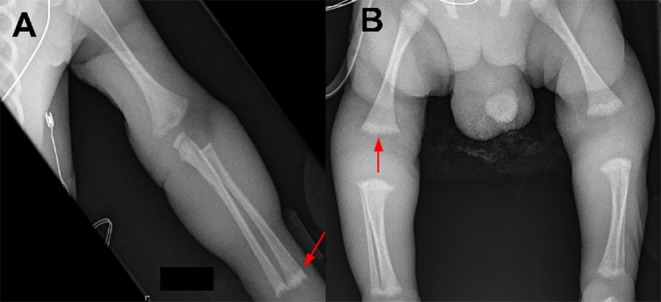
Long bone radiographic findings of a preterm infant with symptomatic congenital syphilis. (A) Sclerotic bands of the metaphyses (arrow). (B) Serrated epiphyseal plates (arrow).
